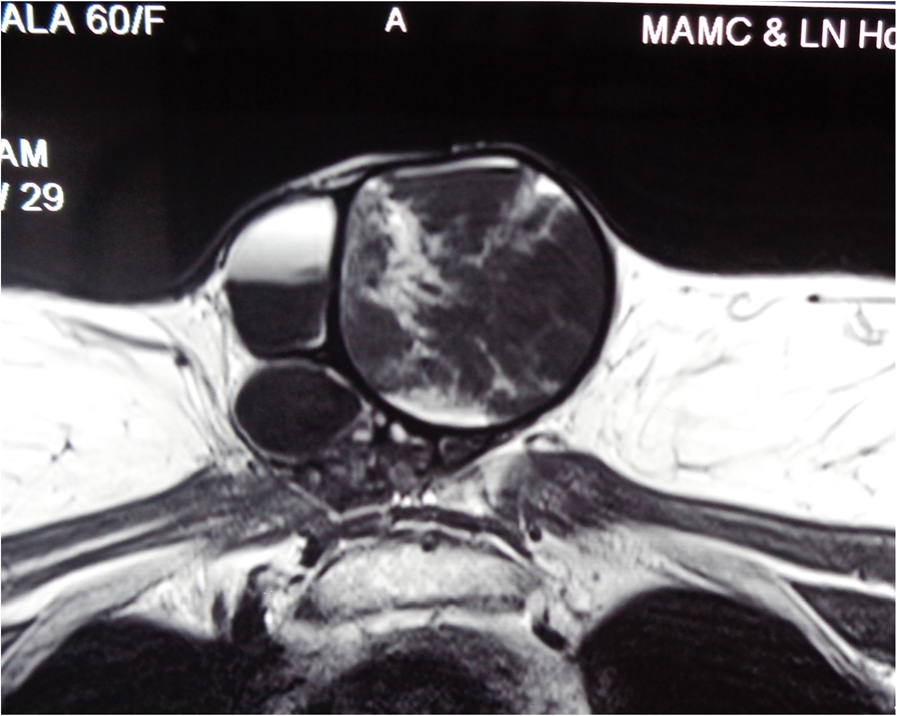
MRI coronal view showing large pre-sternal mass
